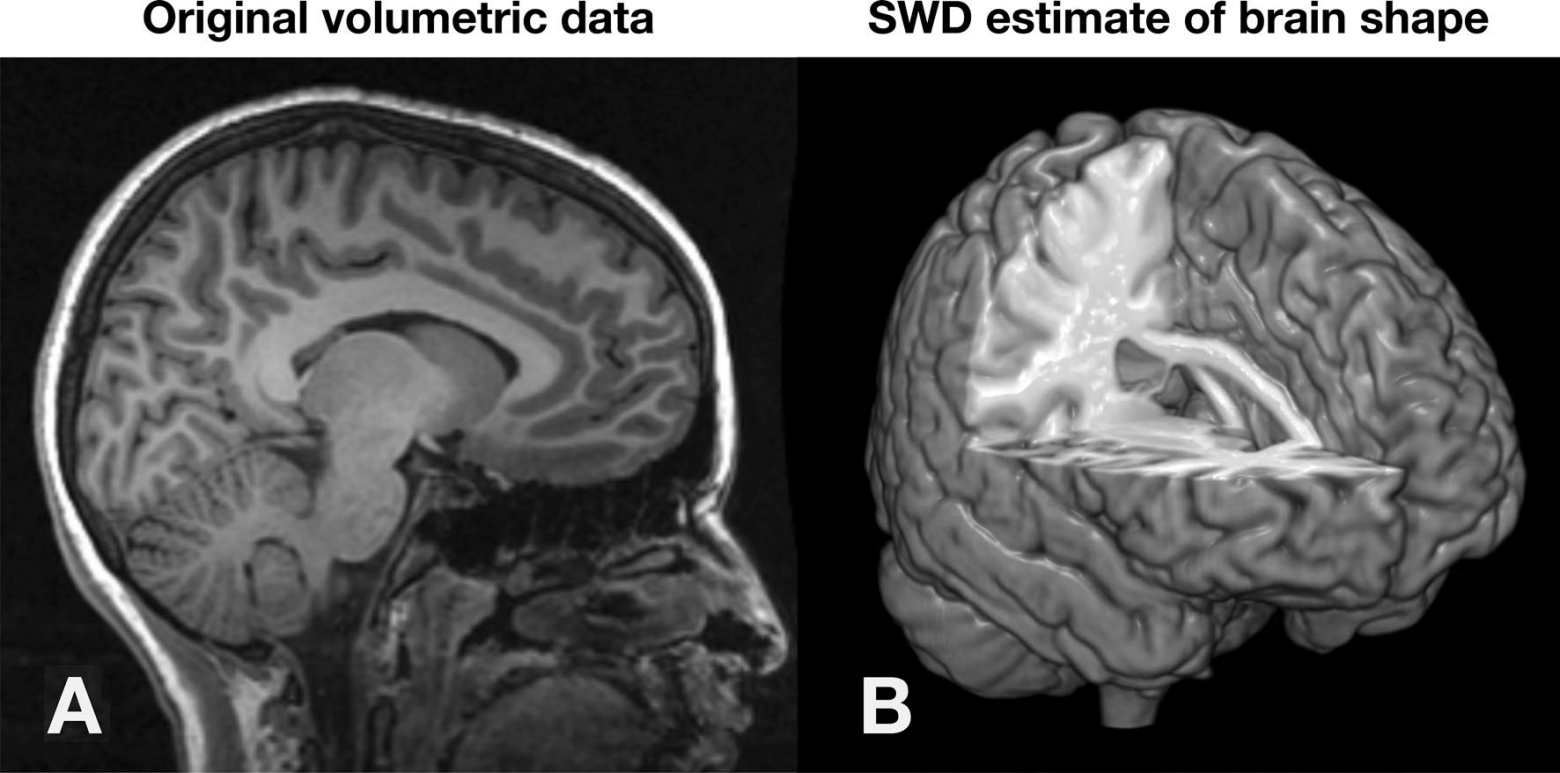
Automated human brain shape characterization by Spherical Wave Decomposition (SWD) from a high resolution anatomical MRI scan. (A) Original volumetric MRI scan, (B) SWD estimate of the brain shape (adapted from^42^). The shape in (B) is characterized by the specific set of $$N=100$$ SWD coefficients, as well as by the number *N* itself, which is calculated as the “optimal” number of coefficients and is thus a measure of the brain shape complexity (see^51^).
